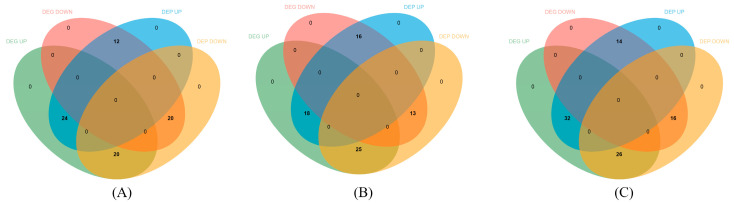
Differentially expressed genes and proteins association analysis in the MT vs. CT (A), HT vs. CT (B), and HT vs. MT (C).
